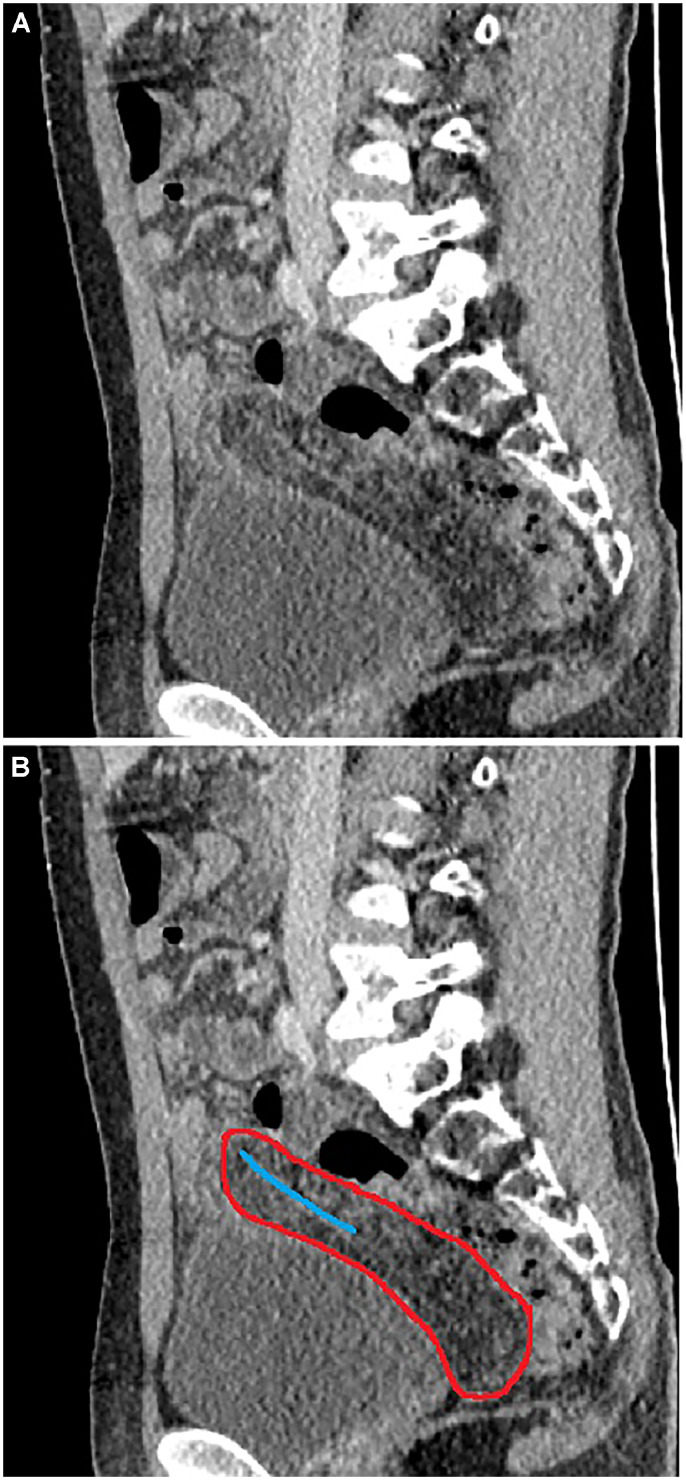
Sagittal contrast-enhanced CT image of volvulus. (A) The red-marked area shows the omental volvulus extending into the Douglas pouch, the blue line indicates the vascular pedicle sign (B).
